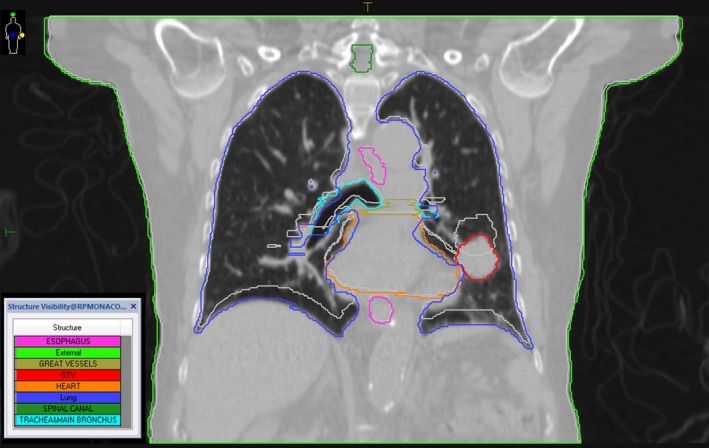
GTV and OAR contours on a coronal section for patient 1 at phase 0% (the colored contours) and phase 50% (the light gray contours). The CT image shown is that of phase 0%.
